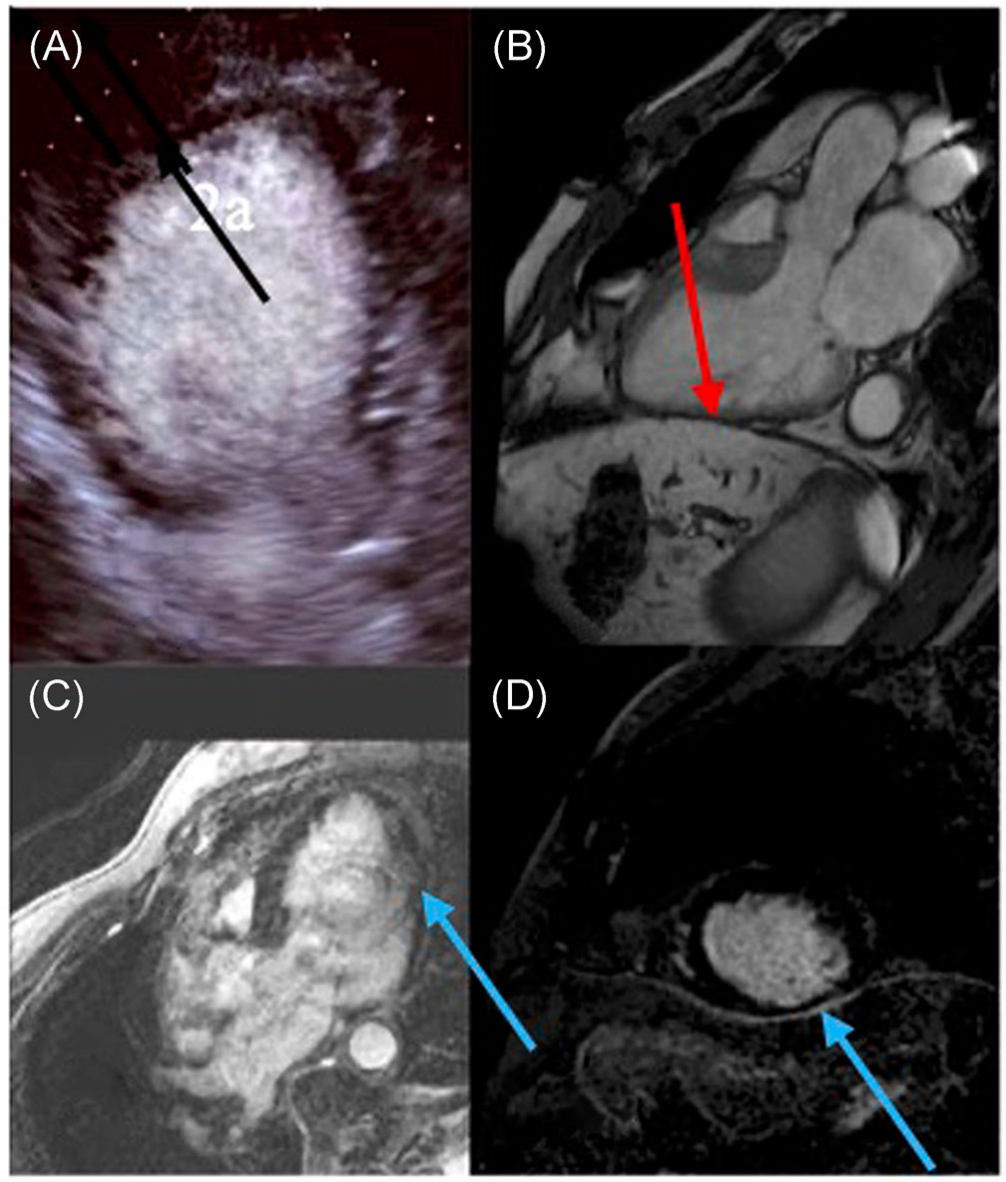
Inferolateral myocardial segment noted as NVM on Echo showed no scar (VM) on CMR. Inferolaterla wall on Echo (A) is thinned with no contractile reserve, suggestive of NVM. Thinned inferolateral wall on CMR (B) was shown to be VM with no LGE (C) and (D). (A) Black arrow: Thinned inferolateral wall on Echo, end diastole. (B) Red arrow: Thinned inferolateral wall on CMR, end diastole. (C) and (D): Blue arrow: Inferolateral wall on CMR with no LGE (scar). CMR, cardiac magnetic resonance; Echo, echocardiography; LGE, late gadolinium enhancement; NVM, nonviable myocardium; VM, viable myocardium
